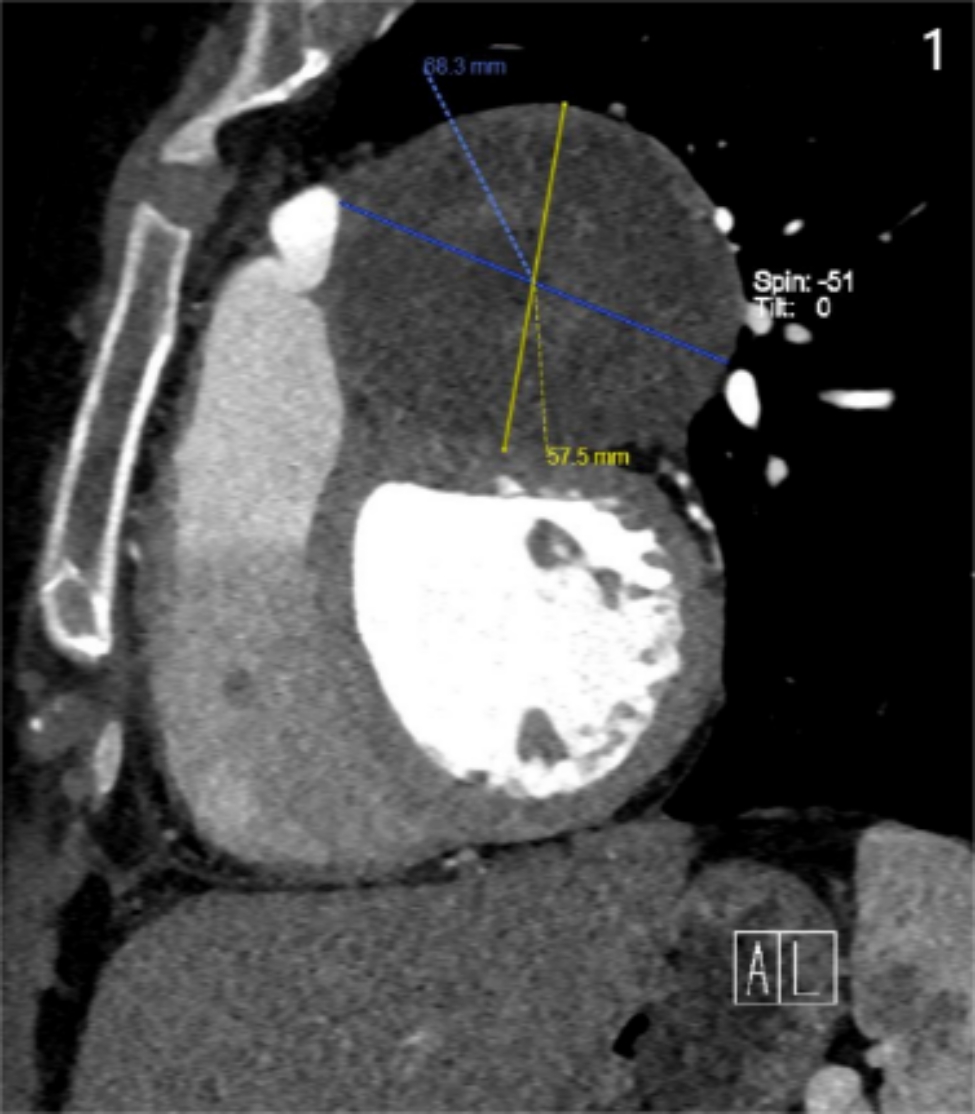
A computed tomographic scan of the coronary artery shows a round-shaped soft tissue density shadow, measuring approximately 6.8 × 5.7 cm
